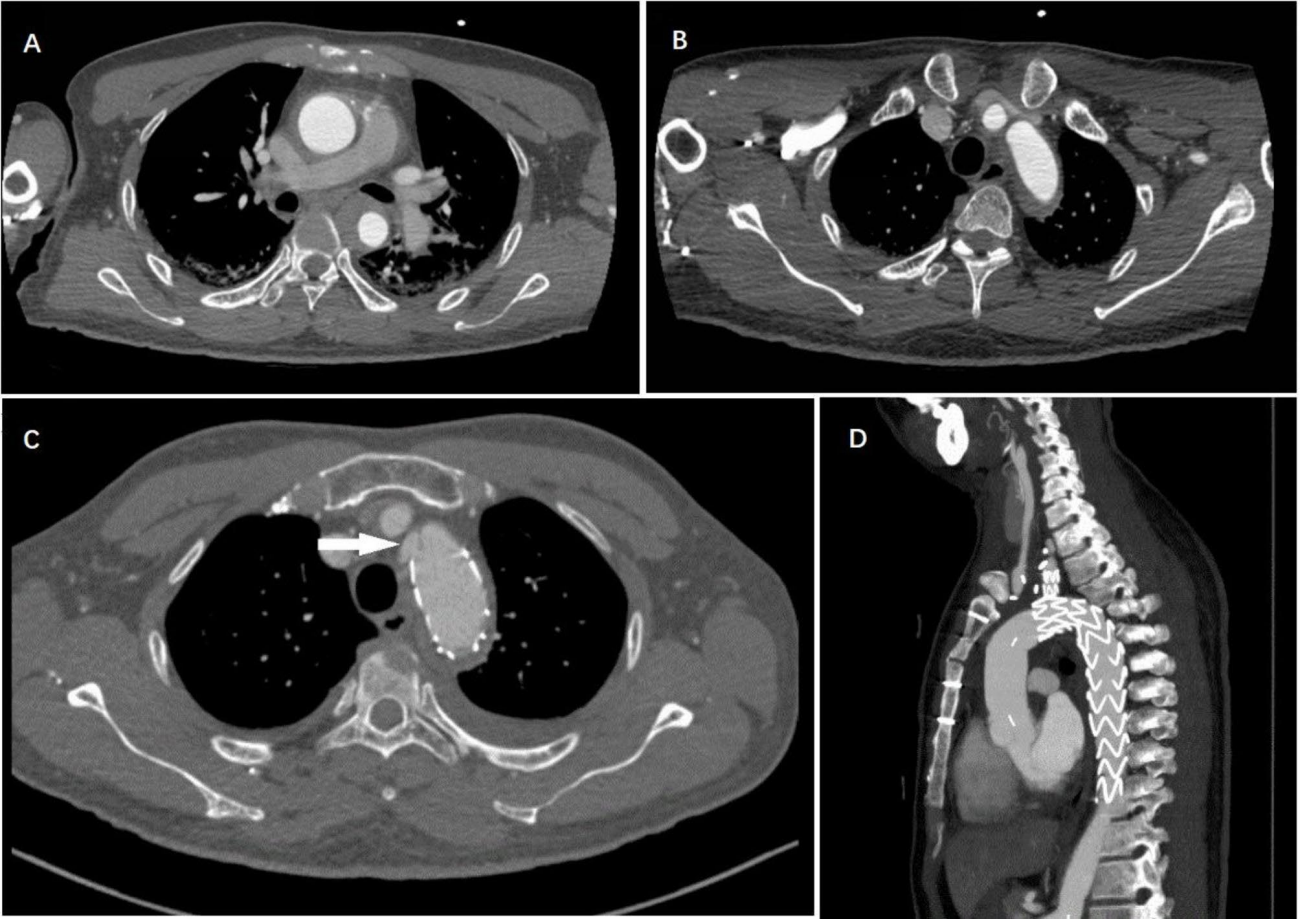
A,B A patient with a Type A intramural hematoma received TEVAR treatment on the first day of onset, and the chest pain persisted after the operation. C 2 weeks after TEVAR, a new CT scan showed a new rupture at the proximal end of the stent (white arrow). D We performed total arch replacement surgery
